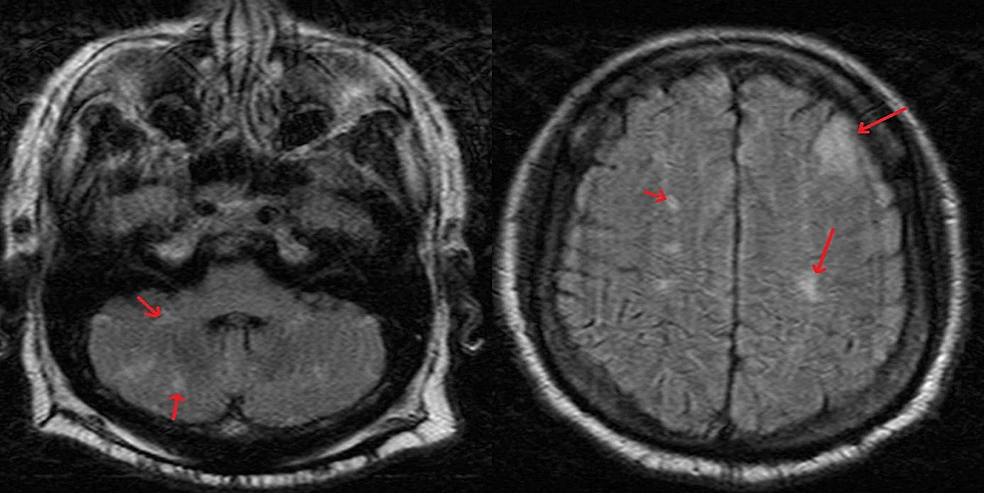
MRI of brain showing acute/subacute bilateral cerebellar hemispheres (left side) and left frontal lobe (right side). MRI, Magnetic resonance imaging.
